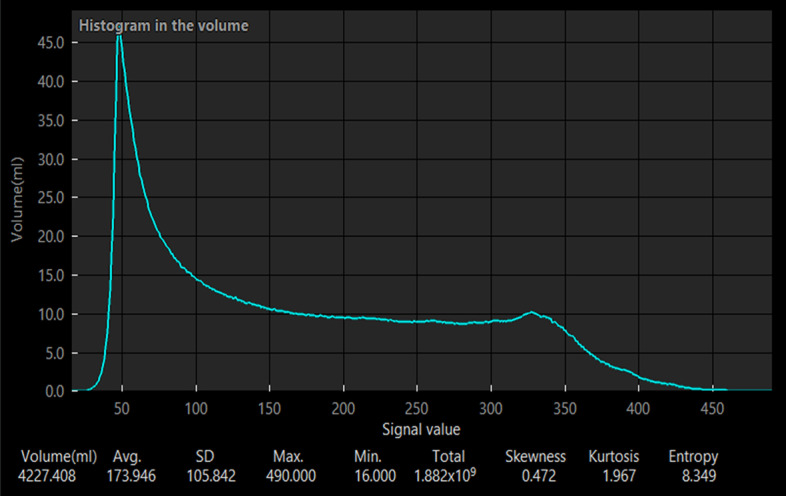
A histogram of radioactivity volume generates from ^99m^Tc-MAA SPECT. The x-axis represents the count from ^99m^Tc-MAA SPECT. The y-axis represents the volume per count. The lung volume was measured as the percentage area with radioactivity higher than the cutoff level of 10% of the maximum count on a histogram. In this case, the count at the edge of the lung perfusion SPECT was 49. Therefore, the total lung volume was calculated by adding up the volume per count from 49 to max count. Abbreviations: ^99m^Tc-MAA SPECT = technetium-99m-macroaggregated albumin single-photon emission computed tomography
